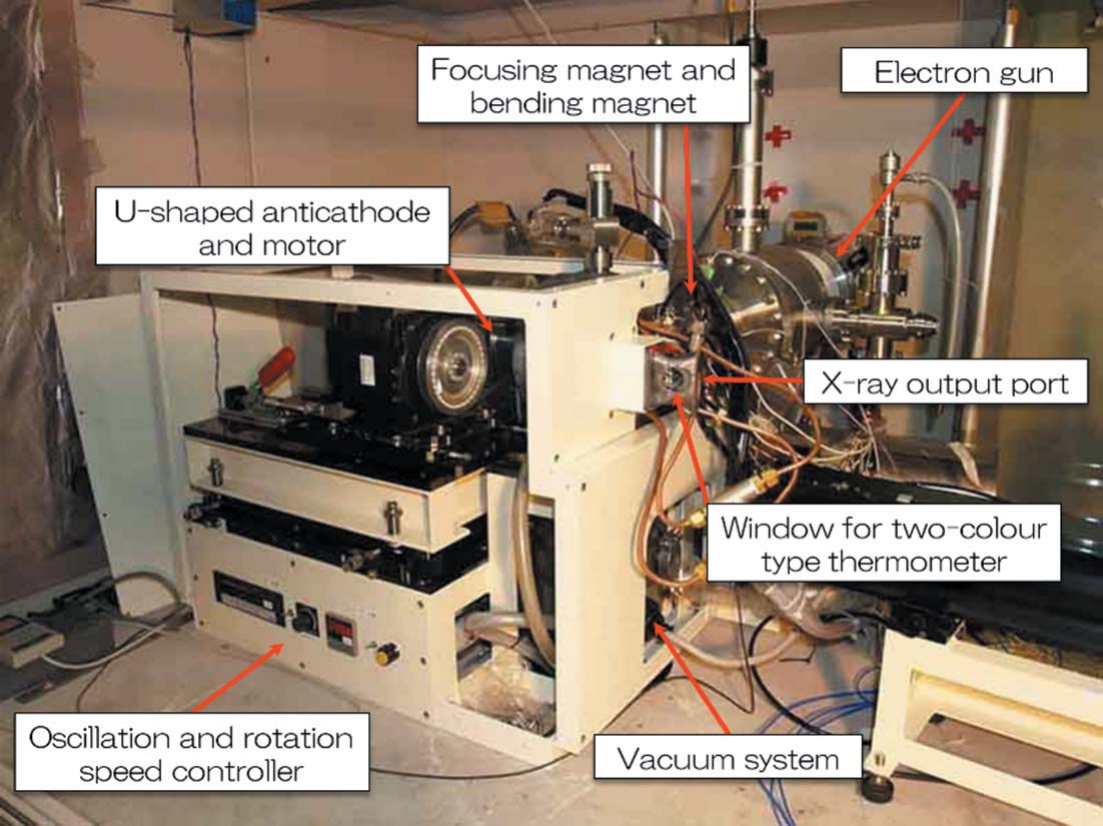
Photograph of the new X-ray generator under test with the U-shaped rotating anticathode. Electrons come from the back on the right and, after being bent horizontally by 180°, irradiate the U-shaped rotating anticathode. X-rays come out in the rightward direction.
